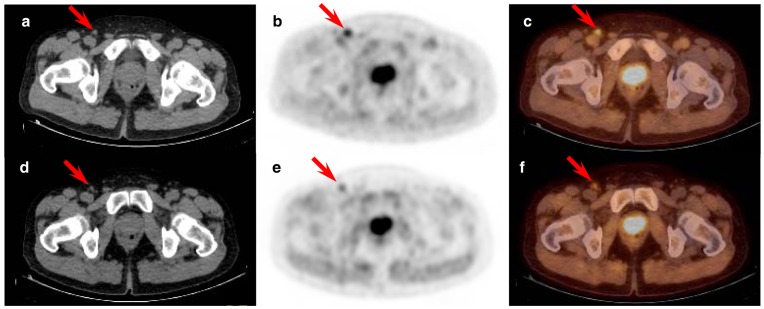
Example of decreasing [18F]FCH uptake over time. Example of decreasing [18F]FCH uptake over time in a right inguinal lymph node (red arrow; SUV_max_ early: 4.41; SUV_max_ late: 2.03) of a patient with newly diagnosed prostate cancer. This lymph node was classified as benign. Transversal sections of the Low-dose CT, PET and fused PET-CT images: a, b and c – early phase; d, e and f – late phase. [18F]FCH uptake in the prostate is also visible.
